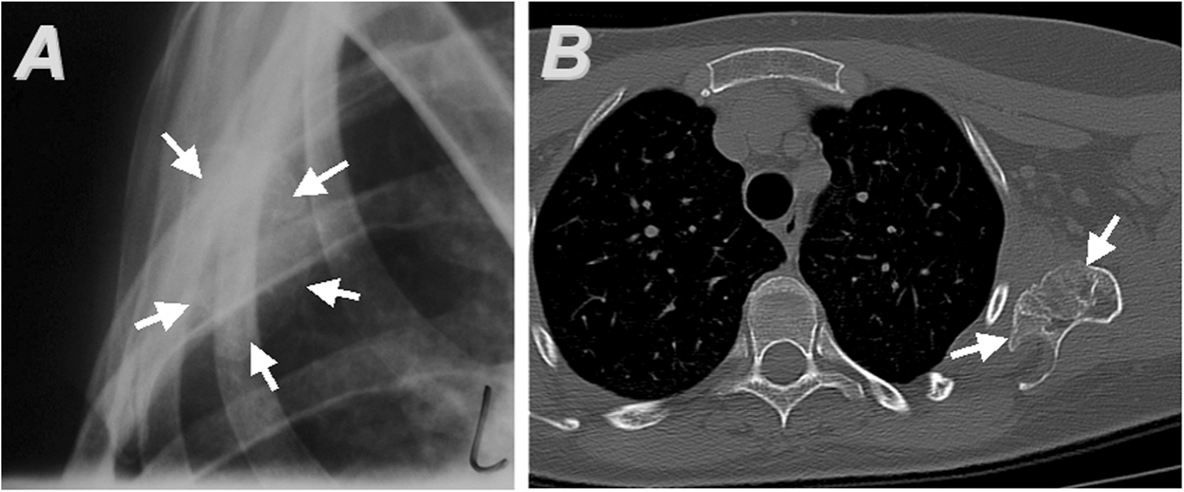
Case 3. (A) A true lateral radiograph of the left scapula shows the tumor arising from the anterior scapular surface. Its margins are indicated by arrows. The computed tomography scan (B) depicts the osteochondroma (arrows) originating from the ventral surface of the scapula, surrounded by the accompanying bursa.
